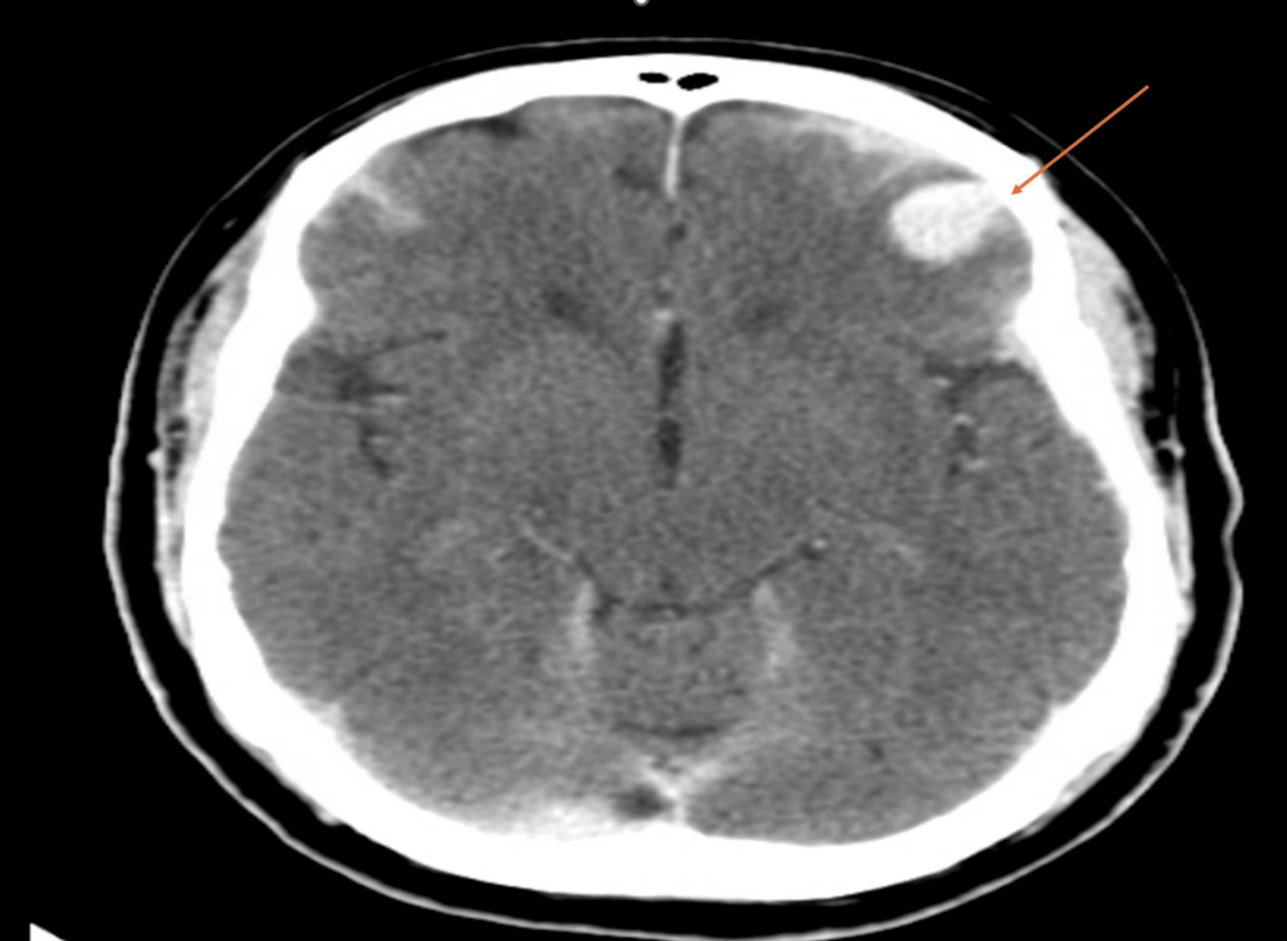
Initial CT of the head showing traumatic hemorrhage (arrow) Arrow highlights acute traumatic intracranial hemorrhage involving subarachnoid and subdural components.
